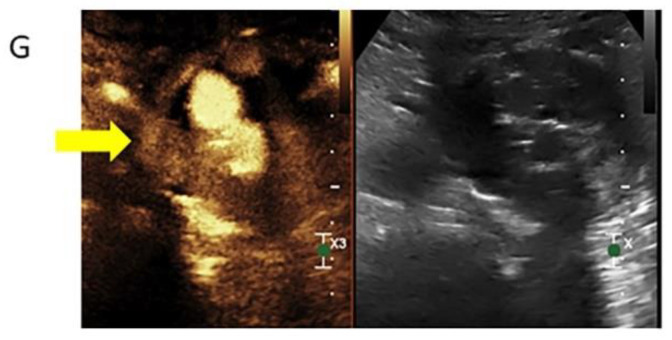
Coronal MIP image (A) demonstrating an infrarenal AAA in a 78-year-old male obtained prior to endovascular repair. Calcific atherosclerotic plaque and vessel patency well-demonstrated on this arterial phase CTA. 100 mL of Omnipaque 350 contrast was administered with CTDIvol of 7.68 mGy. Arterial phase source axial image (B) from a CTA demonstrating endovascular repair of the infrarenal AAA with persistent contrast flow into the excluded aneurysm sac, consistent with an endoleak. Sagittal (C) and coronal (D) MIP images are also shown. Lack of dynamic imaging limits classification of the type of endoleak; although, it was initially characterized as a type II endoleak by CTA. 100 mL of Isovue 370 contrast was administered with CTDIvol of 5.77 mGy. Subsequent contrast-enhanced ultrasound (CEUS, (E)) with dynamic contrast enhancement demonstrated a type III endoleak arising from the origin of the left iliac limb ((F), yellow arrow). The type III endoleak represents the major component and a large portion of the bolus empties into the aneurysm sac ((G), yellow arrow) with a small type II contribution arising from a right lumbar artery (not shown). Three boluses totaling 5 mL of Lumason were injected.
